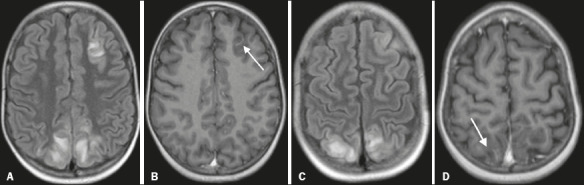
Brain MRI. Axial T2-weighted FLAIR sequences (A and C) and gadolinium
